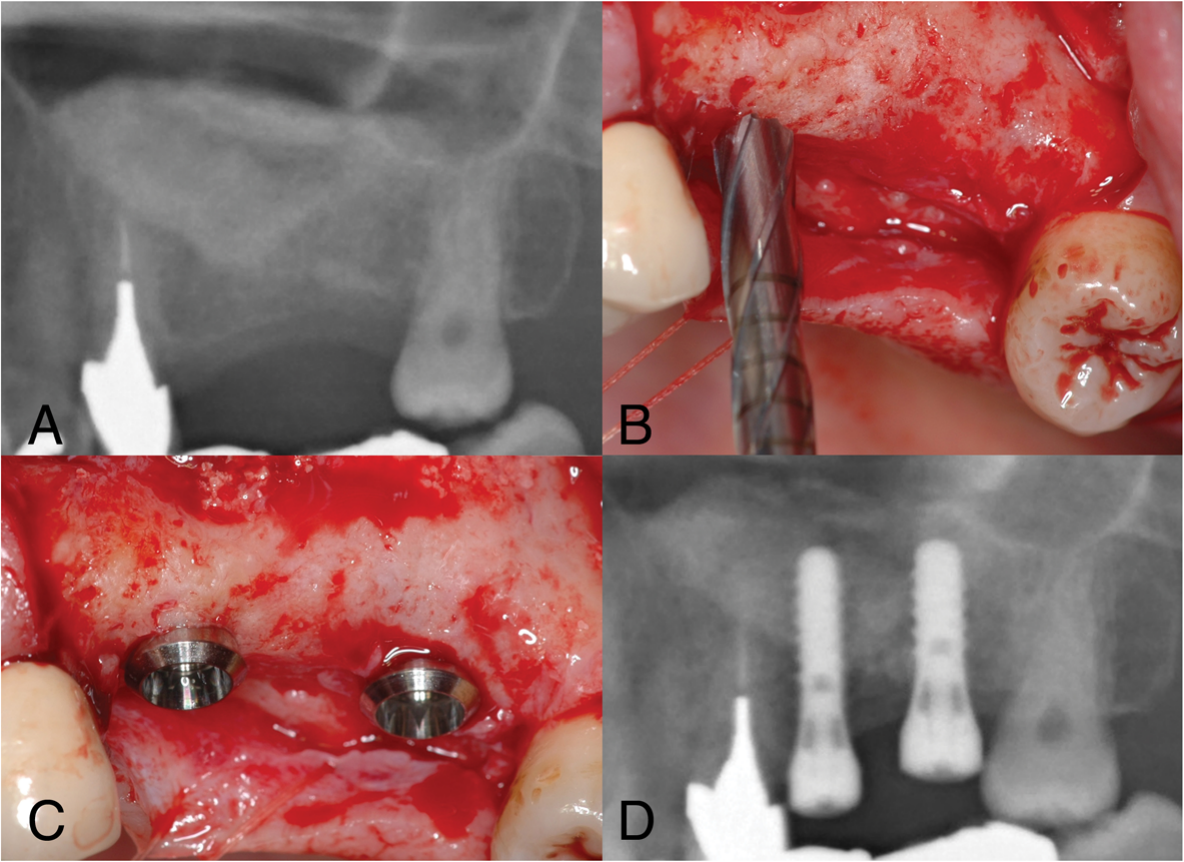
Images of patient # 5 (9-month healing time). a. Radiograph of the left maxillary sinus: situation 9 months after the maxillary sinus floor elevation procedure. b. With a trephine drill, the implant osteotomy is made and the biopsy is obtained. c. Clinical situation after placing two Straumann® SLA implants in the left posterior maxilla. d. Radiograph of two Straumann® SLA implants in the left posterior maxilla
